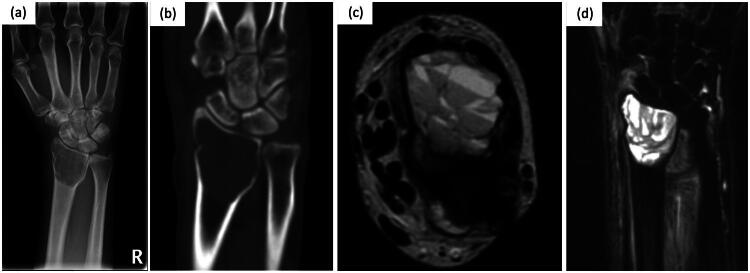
Radiograph of the anteroposterior view (a) at presentation. Coronal computed tomography (CT) image shows cortical bone thinning and microfractures (b). Axial T2-weighted image (c) and T2 fat-saturated sequence (d) show a cystic lesion and fluid-fluid level.
